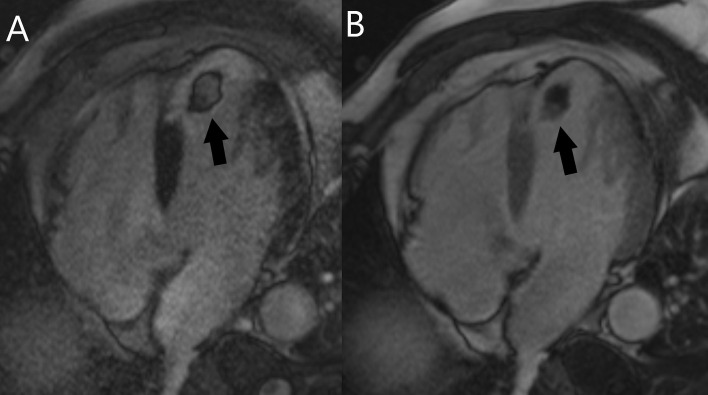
MRI late-gadolinium enhancement in a patient with myocardial
